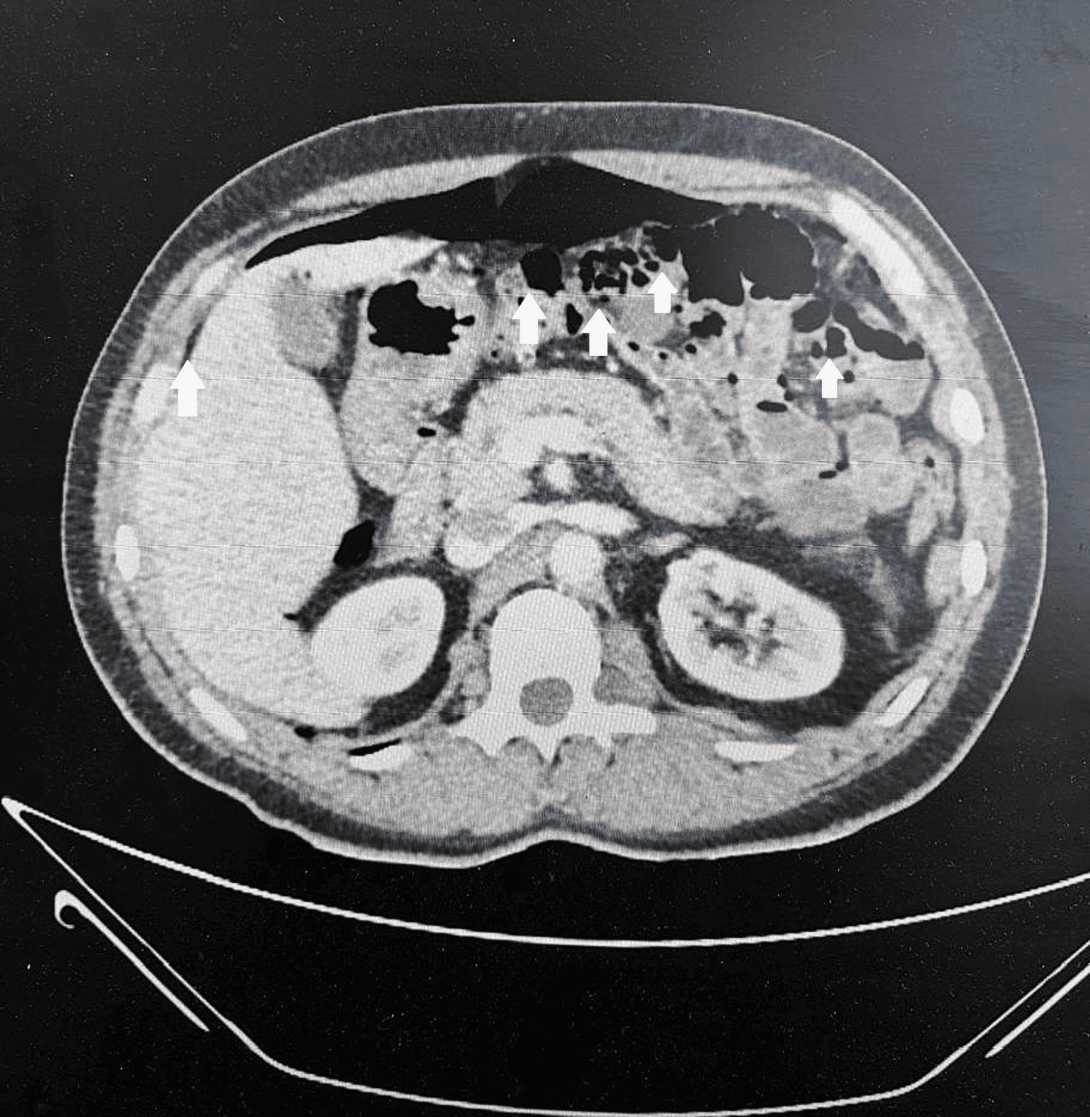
Transverse abdominal CT scan with IV contrast showing pneumoperitoneum, with multiple small bowel diverticula with pneumatosis.
